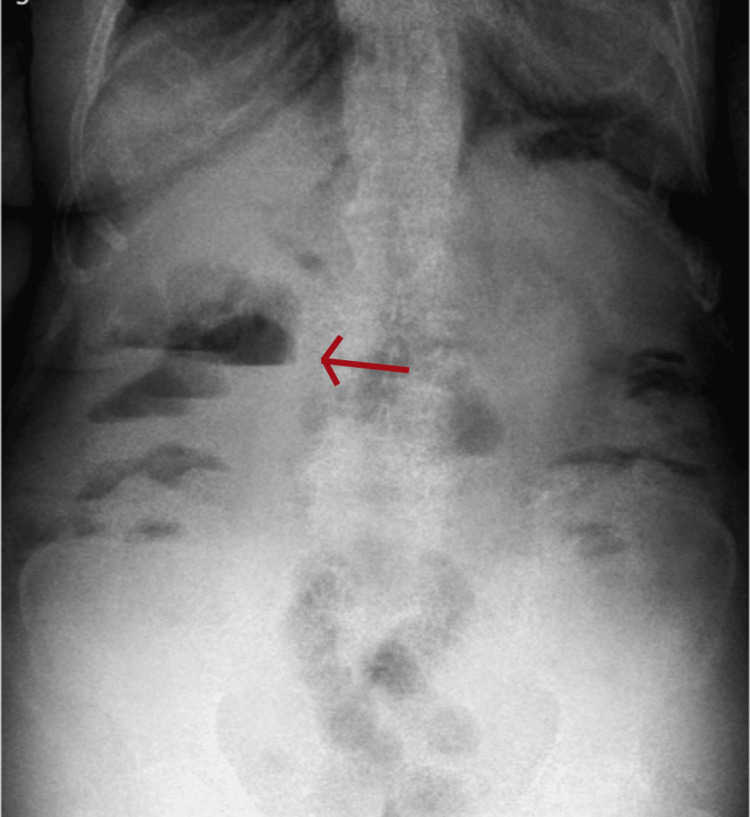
Plain abdominal radiograph in the upright position. Dilatation of small bowel loops with multiple air-fluid levels (red arrow).
